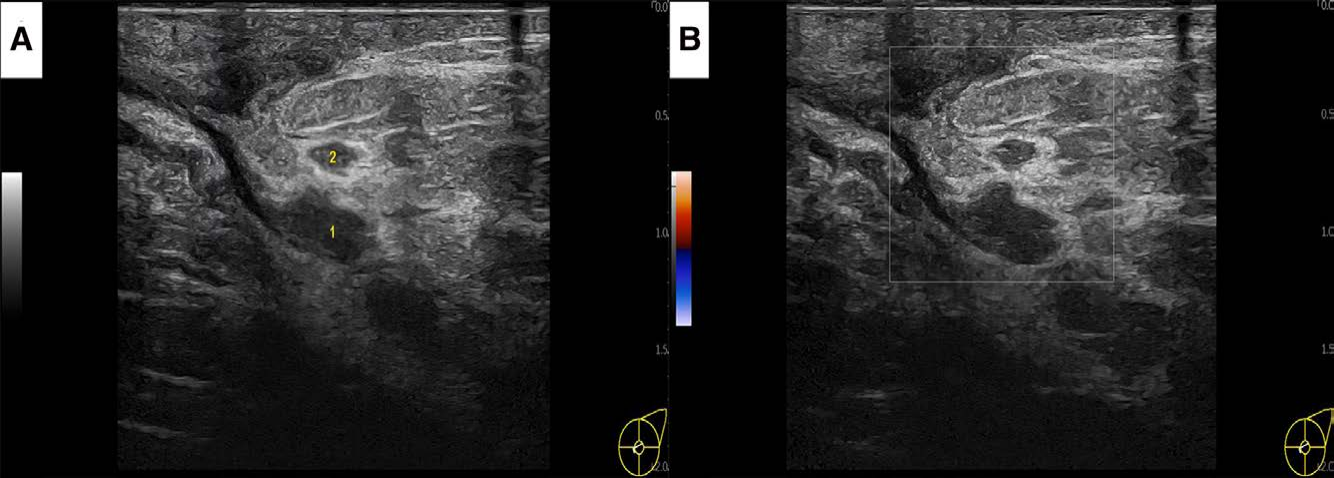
Parapillary abscess located at the 2 to 3 o’clock position on the left breast. (A) The abscess has diminished in size, appearing as multiple low-echo areas, with 1 patch measuring approximately 10 mm by 7 mm; (B) CDFI showed no significant blood flow within the lesion. CDFI = color Doppler flow imaging.
